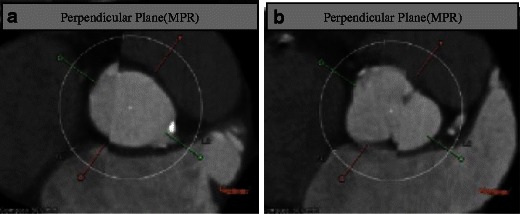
Suboptimal imaging of the aortic annulus in a patient with irregular high heart rate. An 85-year-old female with rapid atrial fibrillation. Due to the irregular heart rate, substantial image registration artefacts severely degraded image quality of CT raw data as well as subsequent post-processing images. Thus, all the required measurements—(a) aortic annulus and (b) width of the SOV, cannot be reliably assessed
